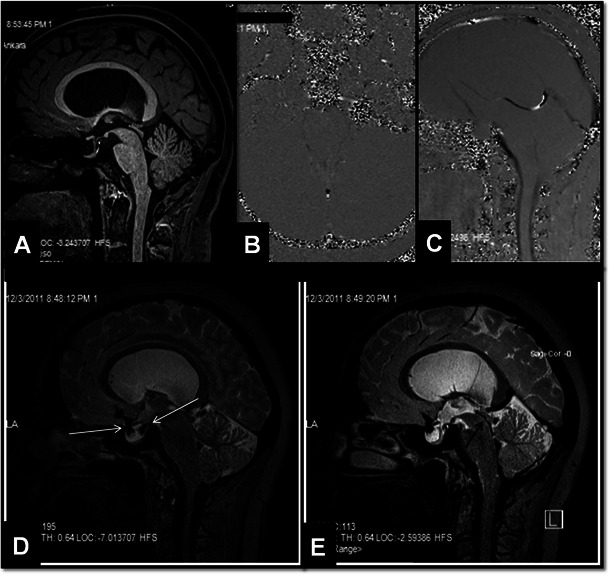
A 32-year-old female patient with partial aqueductal stenosis, partial empty sella and hydrocephalus. In the sagittal midline 3D-MPRAGE image no increase in third ventricle size and no aqueductal abnormalities are detected (A). However, axial and sagittal PC-MRI images (VENC value: 6 cm/s) demonstrate lack of CSF flow in the aqueduct (B, C). Sagittal 3D-SPACE image demonstrates passage of CSF from basal-prepontine cisterns into the sellar cavity through the diaphragm sella (*white arrows*, D). This finding explains why hydrocephalus may be associated with empty sella in most of the patients. In the midline sagittal 3D-SPACE image, a narrowed but patent aqueduct is demonstrated (*black arrow*, E)
